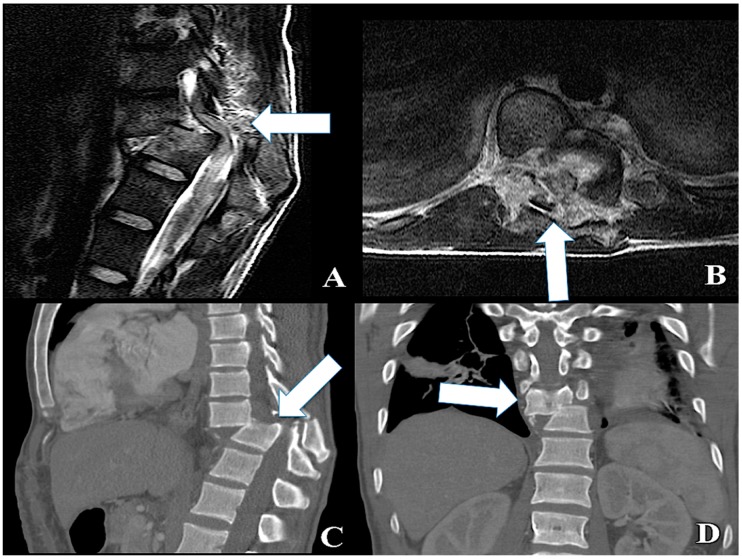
In (A) and (B): a “C”-type Spine Fracture Dislocation with a complete Spinal Cord Injury (arrow). In (C) and (D): a CT scan of the spine disclosing a severe dislocation both on coronal and sagittal plane (arrow).
